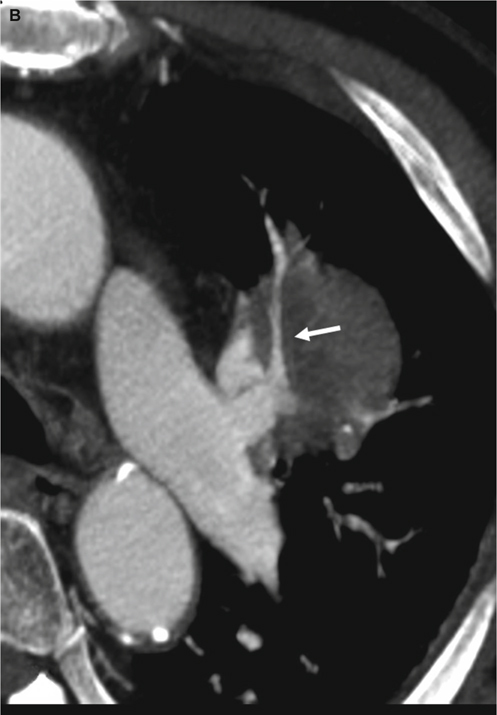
Encasement. This is an 82-year-old male with a mass lesion abutting the left hilum. He received bronchoscopic biopsy and pathology revealed small cell lung carcinoma. Coronal (A) and axial (B) section computed tomography (CT) images reveal the main tumor envelops the left segmental pulmonary artery, with decrease in the diameter of this artery (white arrows).
